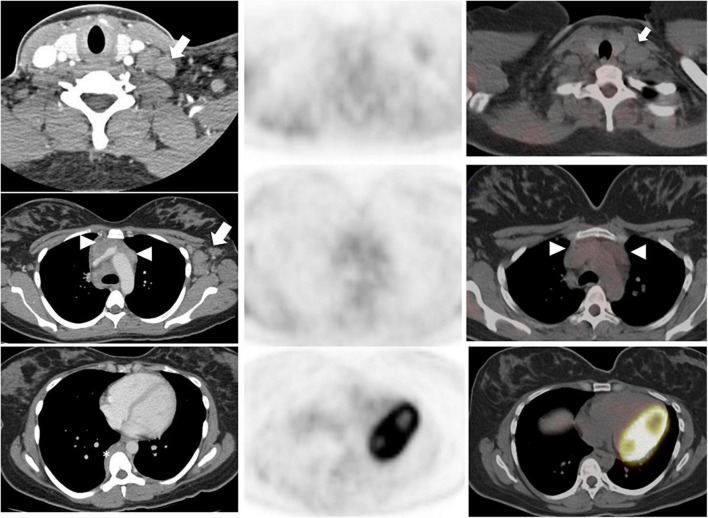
A 28-year-old woman with Stage IIB nodular sclerosing Hodgkin’s lymphoma. Baseline staging with contrast-enhanced CT (left column) showed multiple lymphadenopathies in the left neck, supraclavicular fossa (arrow, top row) and axilla (arrow, middle row), an infiltrative soft tissue mass in the anterior mediastinum (white arrowhead, middle row), and a paravertebral soft tissue lesion in the mid thoracic spine (asterisk, bottom column). Interim ^18^F-FDG PET/CT (PET, middle column; fused PET/CT, right column) after 2 cycles of chemotherapy demonstrated complete metabolic response and significant morphologic response (right column fused PET/CT images); overall Deauville score, 2. A negative interim PET can support the decision to de-escalate therapy; whereas a positive PET may indicate need for therapy escalation.
